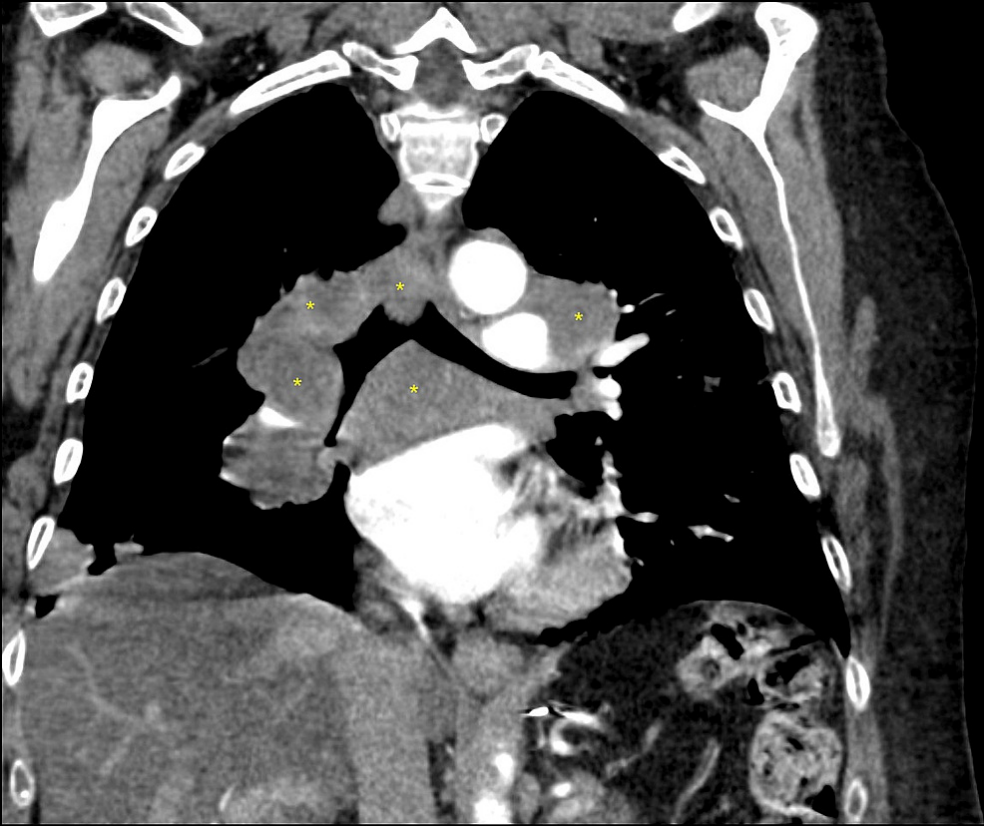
Coronal section of the CT pulmonary angiogram. Bilateral hilar and subcarinal lymphadenopathy causing compression of the right upper lobe, bronchus intermedius, and left upper lobe. Yellow asterisks (*): Enlarged lymph nodes
